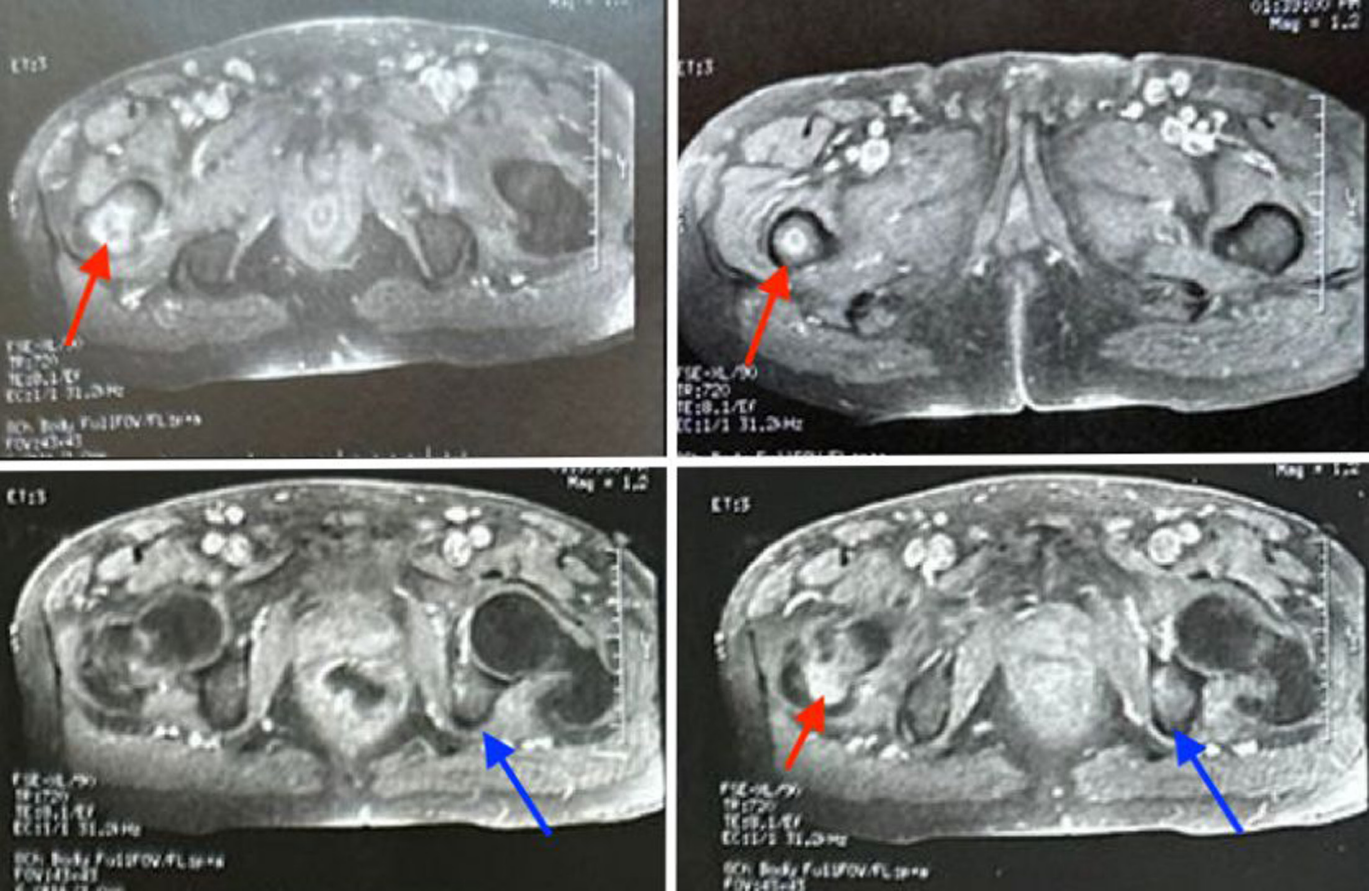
Axial pelvic T1-weighted MRI sequences after gadolinium injection show enhanced intramedullary foci. The red arrow indicates a hyperintense lesion in the right greater trochanter and metaphysis, while the blue arrow highlights a similar lesion in the left iliac bone, both demonstrating post-contrast enhancement consistent with foci of osteomyelitis.
